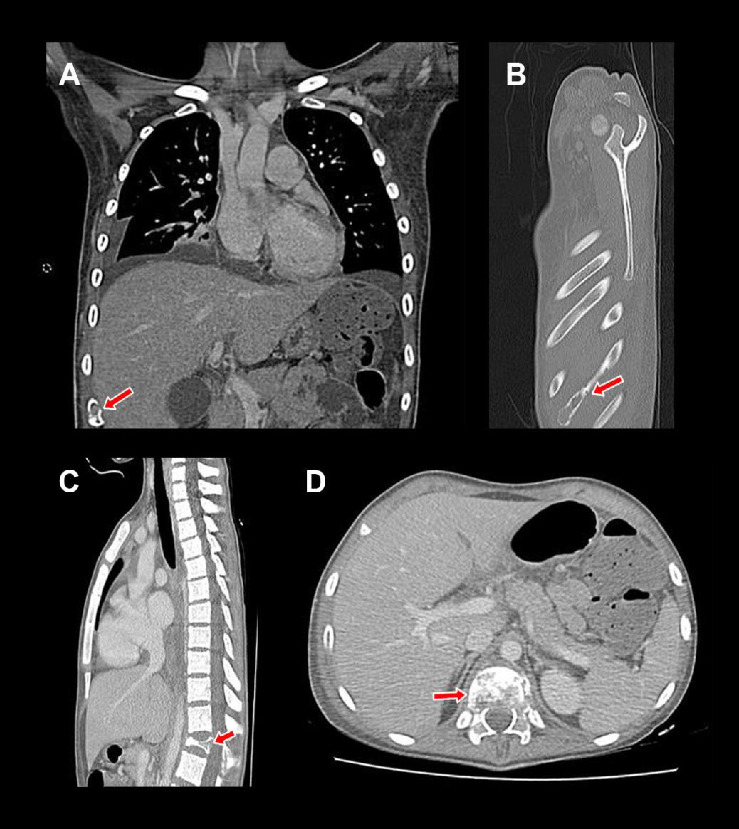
Findings from the CT scan performed upon admission to the emergency department. Lesions identified by the test are denoted by red arrows. The CT scan images revealed osteolytic lesions in the right ninth costal arch, as demonstrated in panels: (A) coronal section and (B) longitudinal section. Additionally, similar lesions were found at the level of the D12 vertebral body, resulting in compression and subsequent collapse of the vertebral body, as shown in panels: (C) longitudinal section and (D) axial section.
